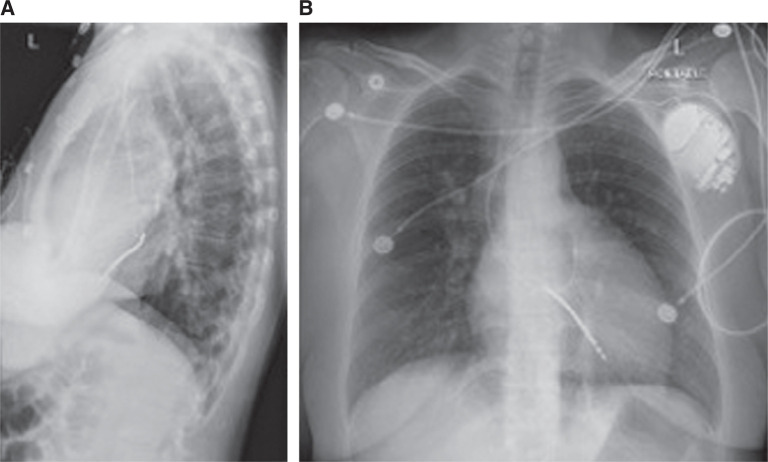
Frontal (right) and lateral (left) chest X-rays showing the single-coil ICD lead terminating in the LV and the atrial lead terminating in the native LAA in the SVA. The cardiomediastinal silhouette is stable and mildly enlarged. There was minimal atelectasis/scarring within the retrocardiac region and mild pulmonary vascular congestion. No obvious pleural effusion or pneumothorax and no acute osseous lesions were observed. Shown here are anteroposterior (A) and lateral (B) views, providing better visualization of the ICD leads.
